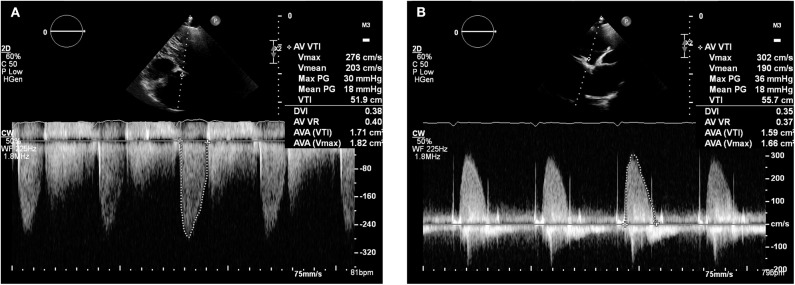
Transthoracic echocardiogram (March 2019): Continuous wave doppler in the apical 5 chamber view (A) and right parasternal long axis view (B) demonstrating a maximum transaortic velocity of 3.0 m/s.
